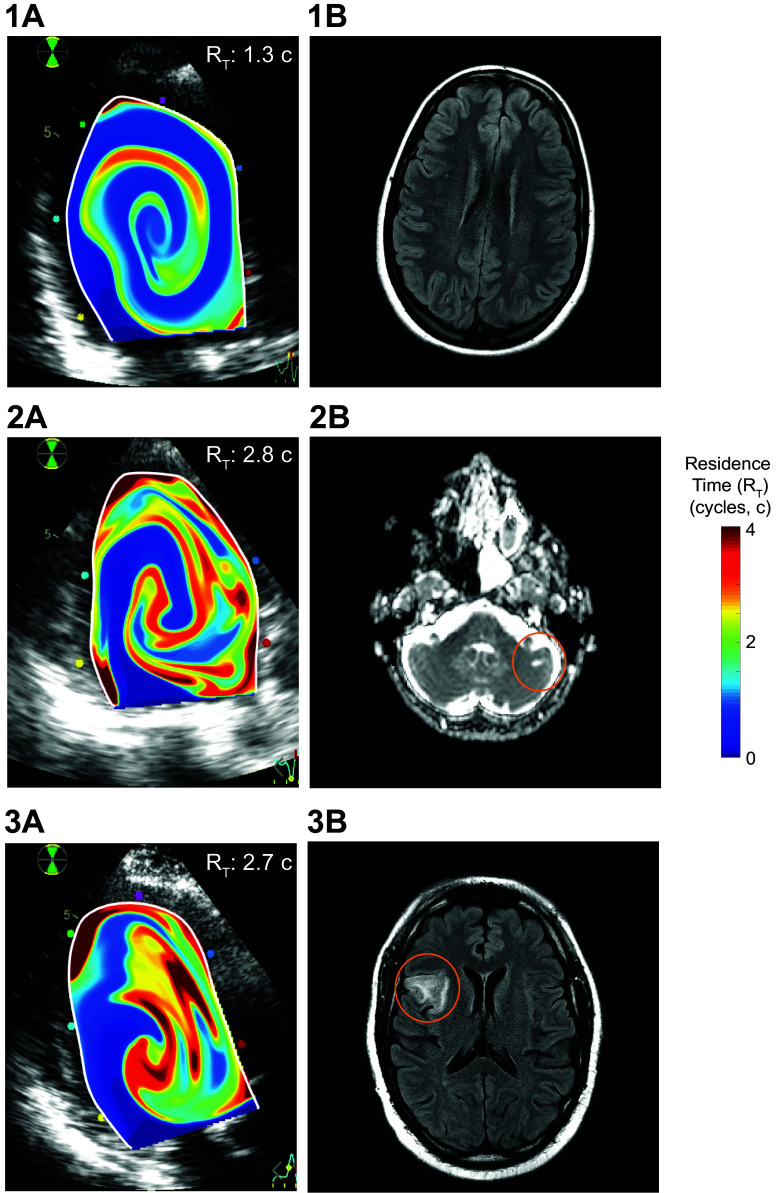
Examples of residence time mapping and brain MR. *1A* and *1B*: residence time (*T*_R_) distribution in the left ventricular (LV) and brain MR of a patient with nonischemic dilated cardiomyopathy (NIDCM) without event. *2A* and *2B* and *3A* and *3B*: *T*_R_ distribution in the LV and brain MR of two patients with NIDCM with a silent brain infarction (SBI, 2*A* and *2B*) and a stroke (3*A* and *3B*). *T*_R_ maps are overlaid on the B-mode echocardiogram.
